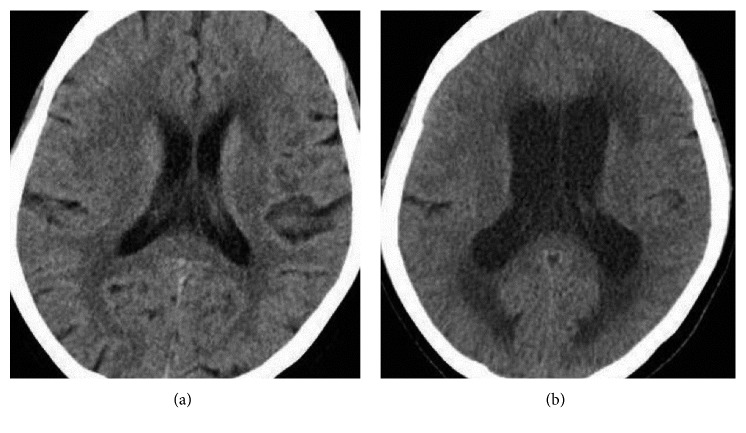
Head CT images at hospital admission (a) and on day 16 (b). The lateral ventricle on day 16 is larger than that at hospital admission.
